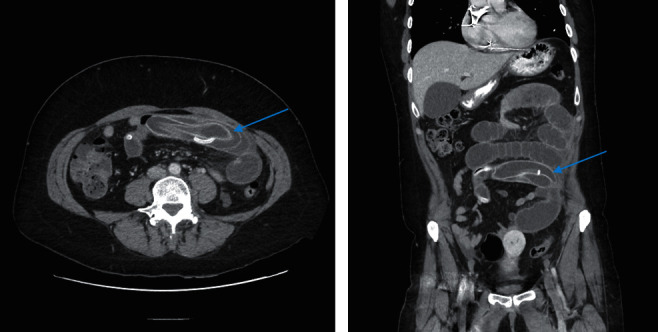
Computed tomography abdomen pelvis, portal venous phase showing small bowel obstruction due to migrated intragastric balloon (blue arrow). (a) Axial view. (b) Coronal view.
